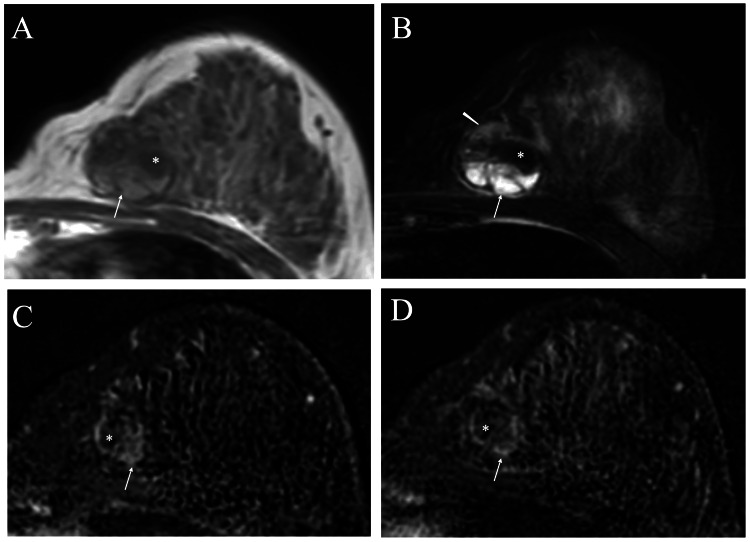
Magnetic resonance image (MRI) findings A. T1-weighted images showed both hypo- (asterisk) and hyper- (arrow) intense areas in the mass. B. Fat-suppressed T2-weighted images showed areas that were hypo-intense (asterisk), slightly hyper-intense (arrowhead), and markedly hyper-intense (arrow). C. Time-signal intensity images showed hypo-intense (asterisk) and hyper-intense (arrow) areas in the mass on early phase images. D. Time-signal intensity images showed that the hypo-intense area (asterisk) remained hypo-intense but the hyper-intense area showed a washout pattern (arrow) on late-phase images.
